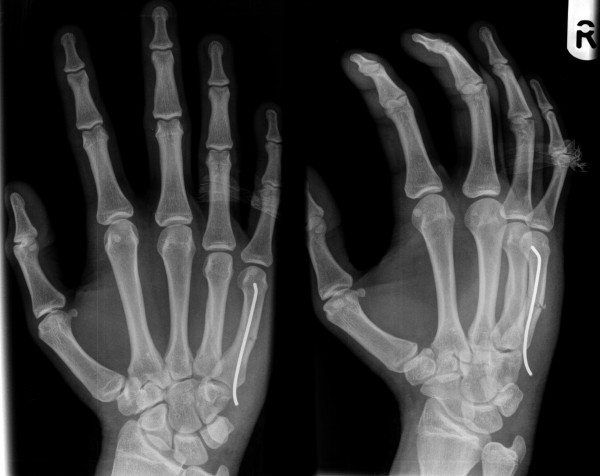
Post-operative radiograph of satisfactory correction of angulation in the diaphyseal fracture.
